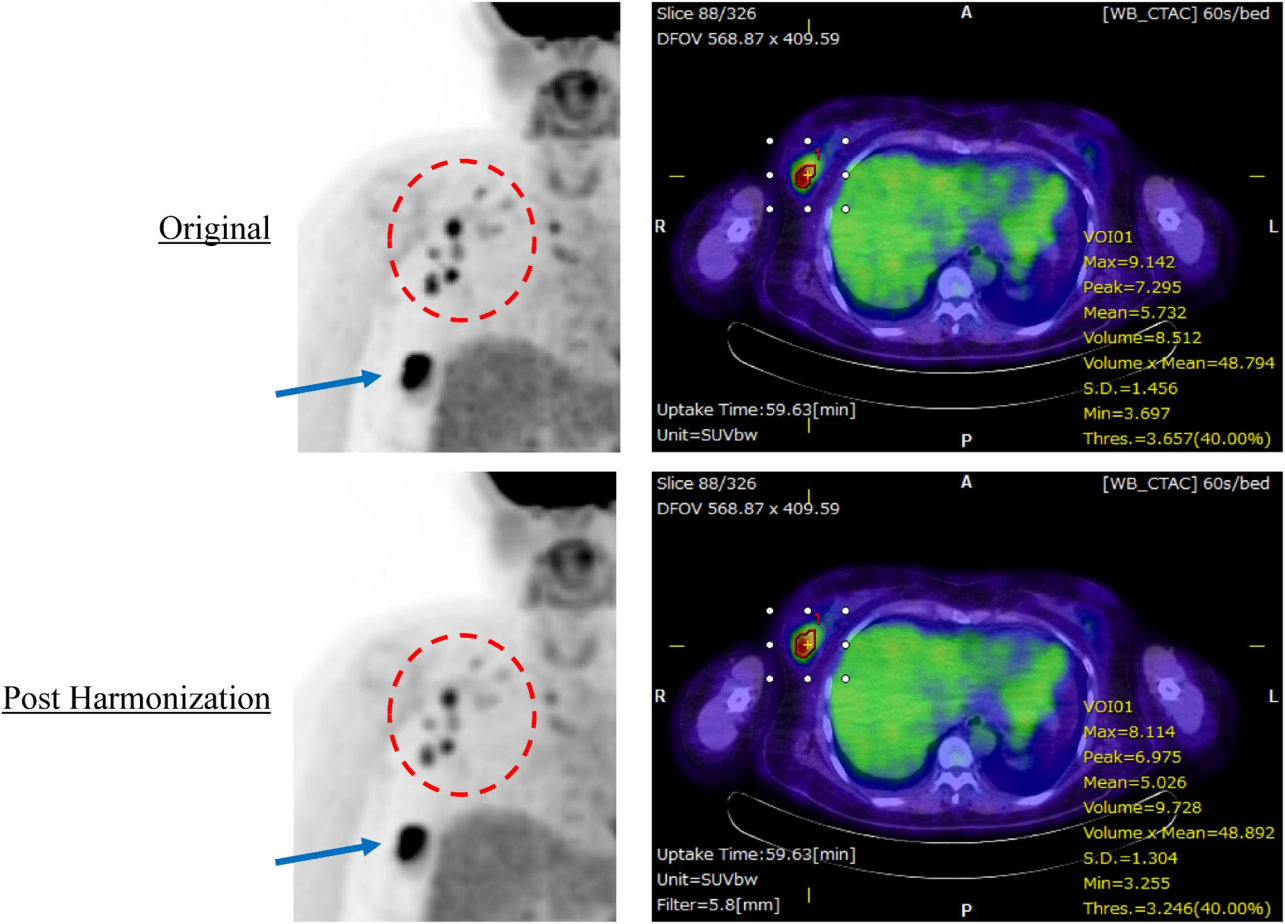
A case with accumulation of FDG in the right primary breast cancer and metastatic lymph nodes. Maximum intensity projection images (left column) and fused PET/CT images (right column) before and after harmonization are shown. The fused images show quantitative values calculated by applying a threshold of 40% to VOI using RAVAT for right primary breast cancer lesions.
